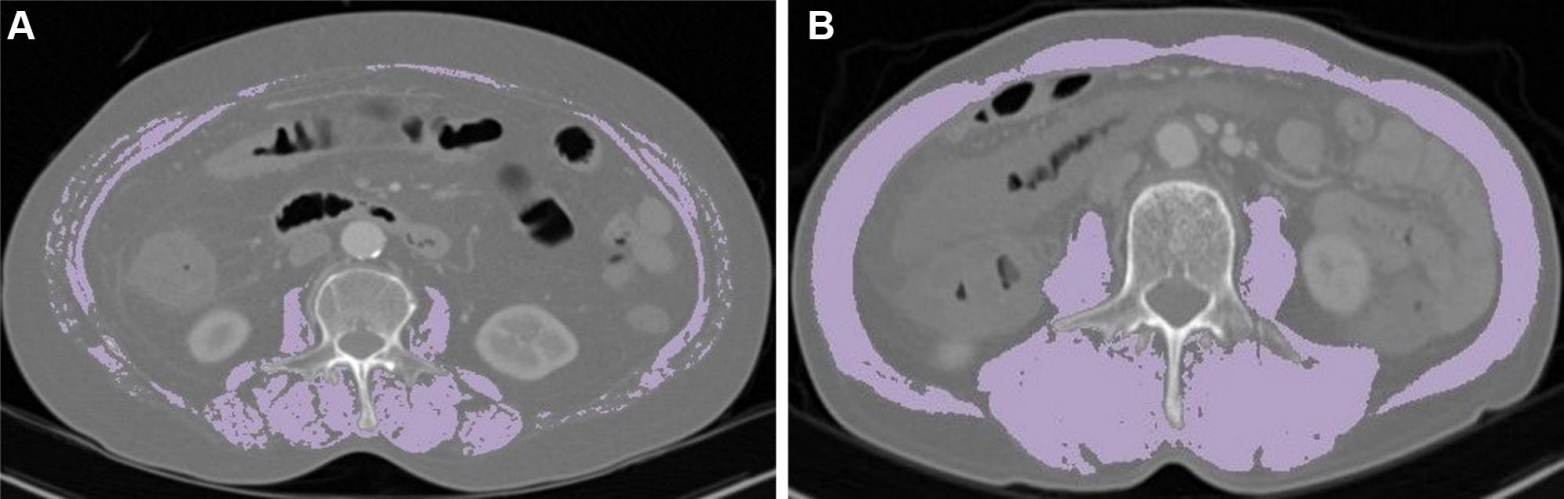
Computed tomography (CT) images of the skeletal muscle area (SMA, shown in bright purple) were obtained at the level of L3 vertebrae in sarcopenic (A) and non-sarcopenic (B) ischemic colitis patients using the "AsanJ-Morphometry" software^22^.
